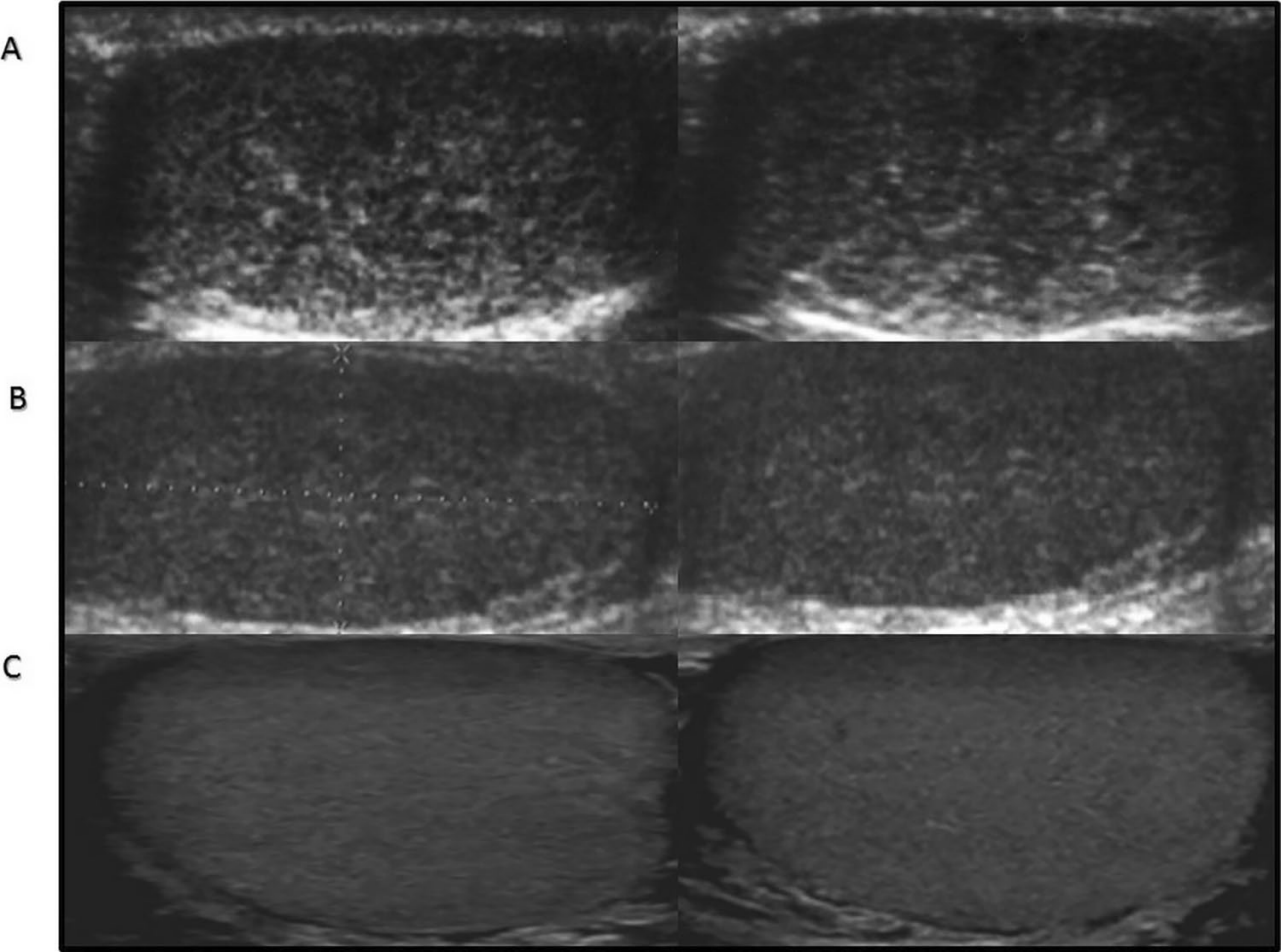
Ultrasound features of testes with TM in pubertal patients. A 16.7 years old, B 13.7 years old, C healthy control 15 years old
